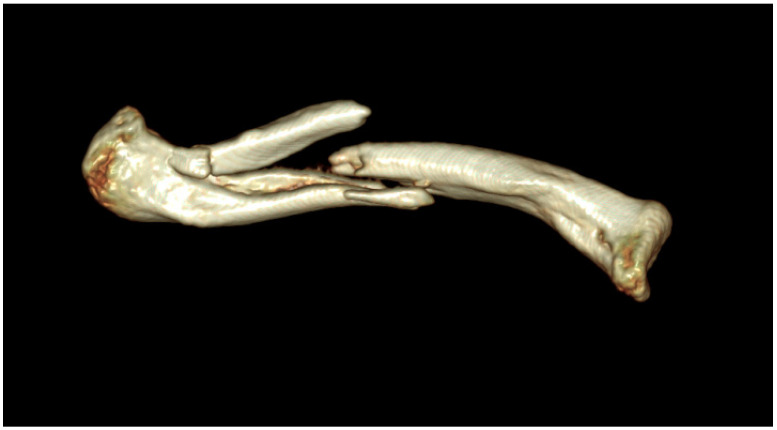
3D-reconstructed CT image of the same patient. Through this image, the pattern of the fracture can be seen more intuitively.
